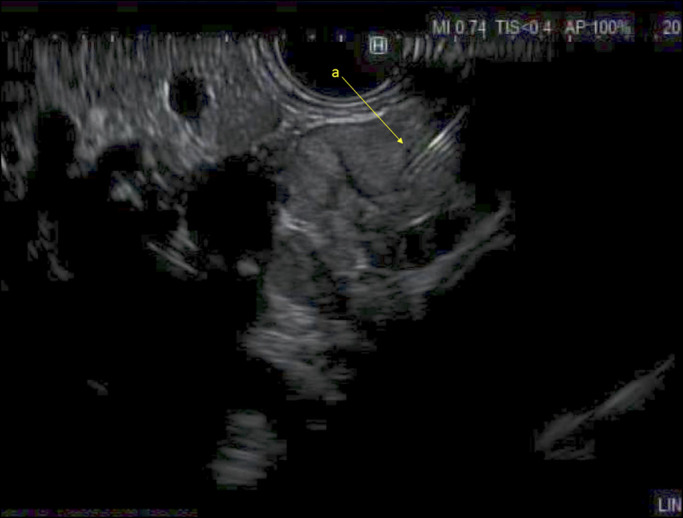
Endoscopic ultrasound-guided fine-needle biopsy of the hypoechoic lesion (a).
